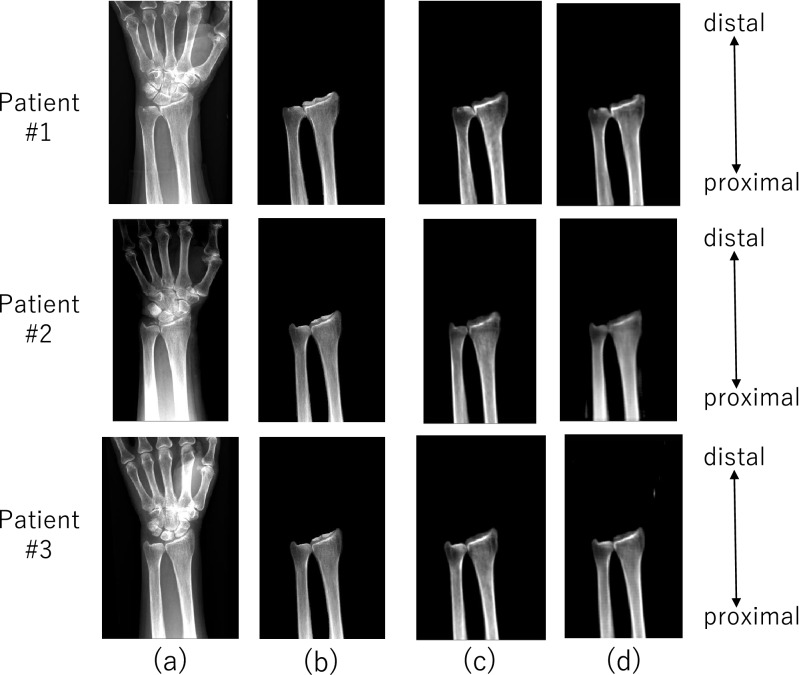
Image translation: (a) actual X-ray image; (b) actual X-ray image showing segmented radius and ulna; (c) registration-DRR; (d) GAN-DRR.
